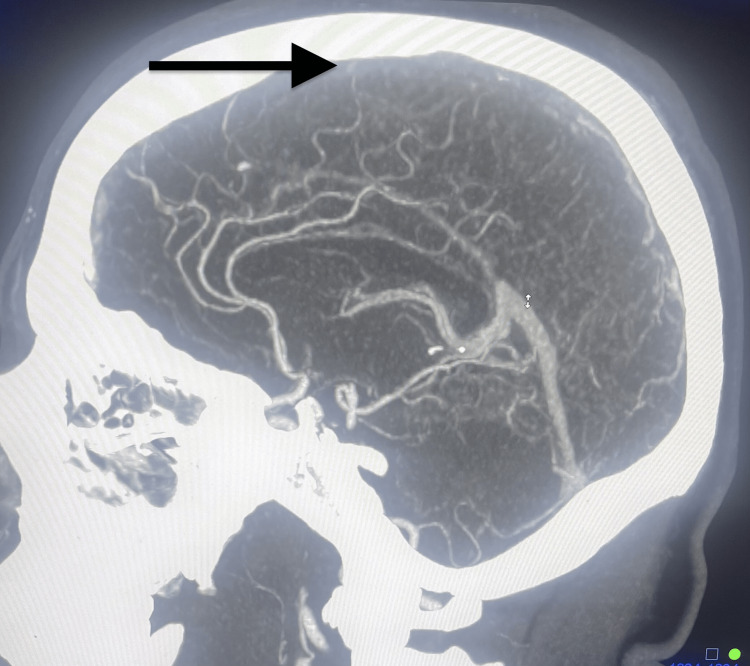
Head CT angiogram The angiogram shows a lack of enhancement in the superior sagittal sinus (arrows) concerning cerebral venous thrombosis.
